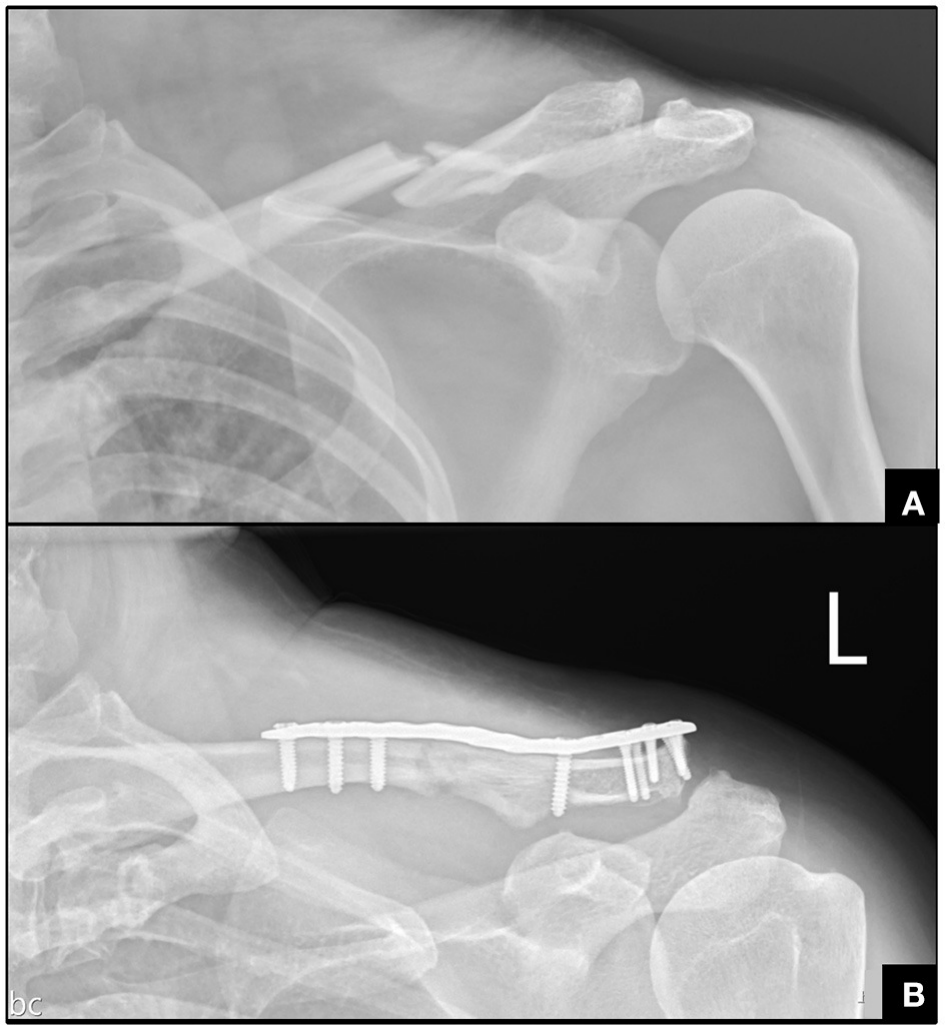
Radiography for the clavicle fracture. (A) Left middle-third clavicle fracture. (B) Postoperative radiography.
